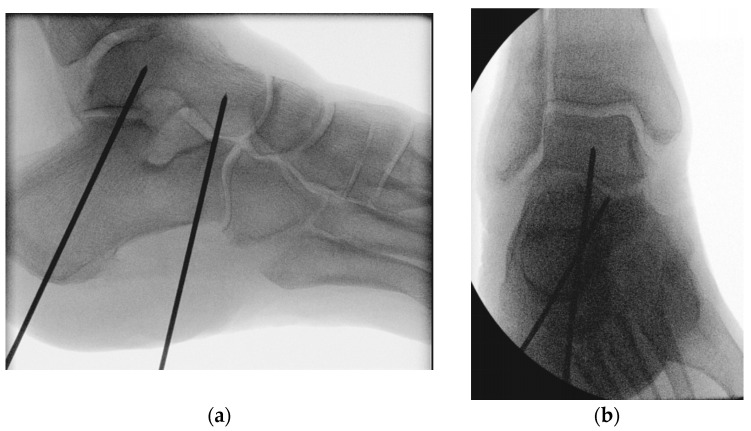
Radiographs visualizing the positioning of the two 2 mm guide wires used for screw insertion with delta configuration during subtalar joint arthrodesis in lateral (a) and anteroposterior (b) views.
